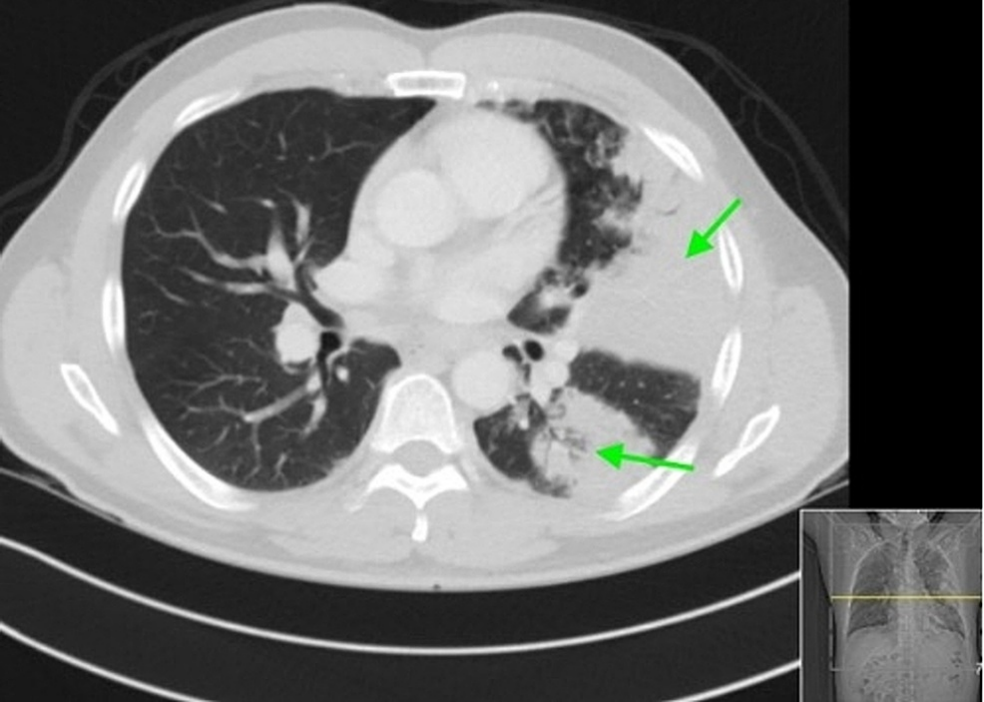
CT showing ground glass airspace opacities bilaterally in combination with dense areas of pulmonary consolidation particularly involving the left upper lobe with the consolidation of at least half of the left upper lobe.
